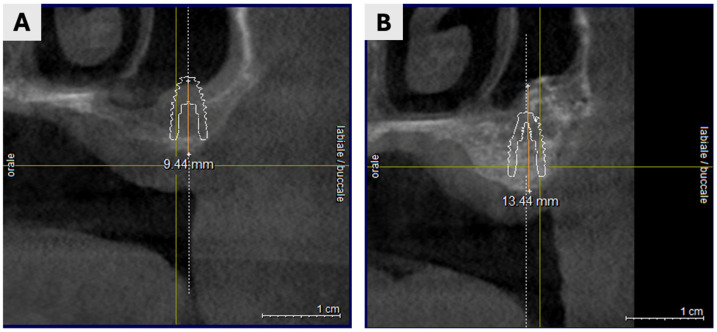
CBCT evaluation of vertical bone height six months after bilateral sinus floor elevation, prior to implant placement. (A) Left maxillary sinus showed vertical bone height of 9.44 mm. (B) Right maxillary sinus showed vertical bone height of 13.44 mm. Both sites demonstrated sufficient vertical bone volume for implant placement, with an intact Schneiderian membrane and no radiographic signs of pathology.
